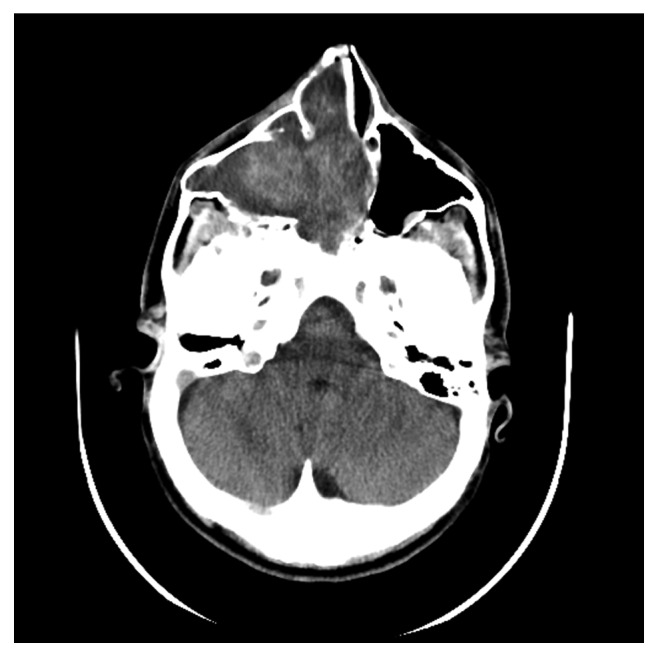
Preoperative CT, axial plane.
